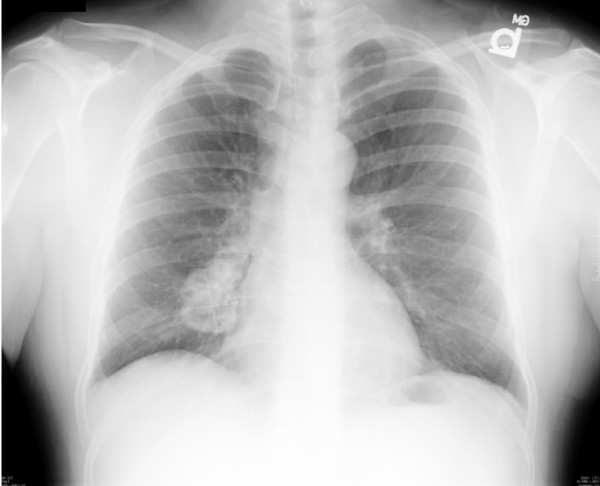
Chest X-ray showing a right infrahilar tumor.
